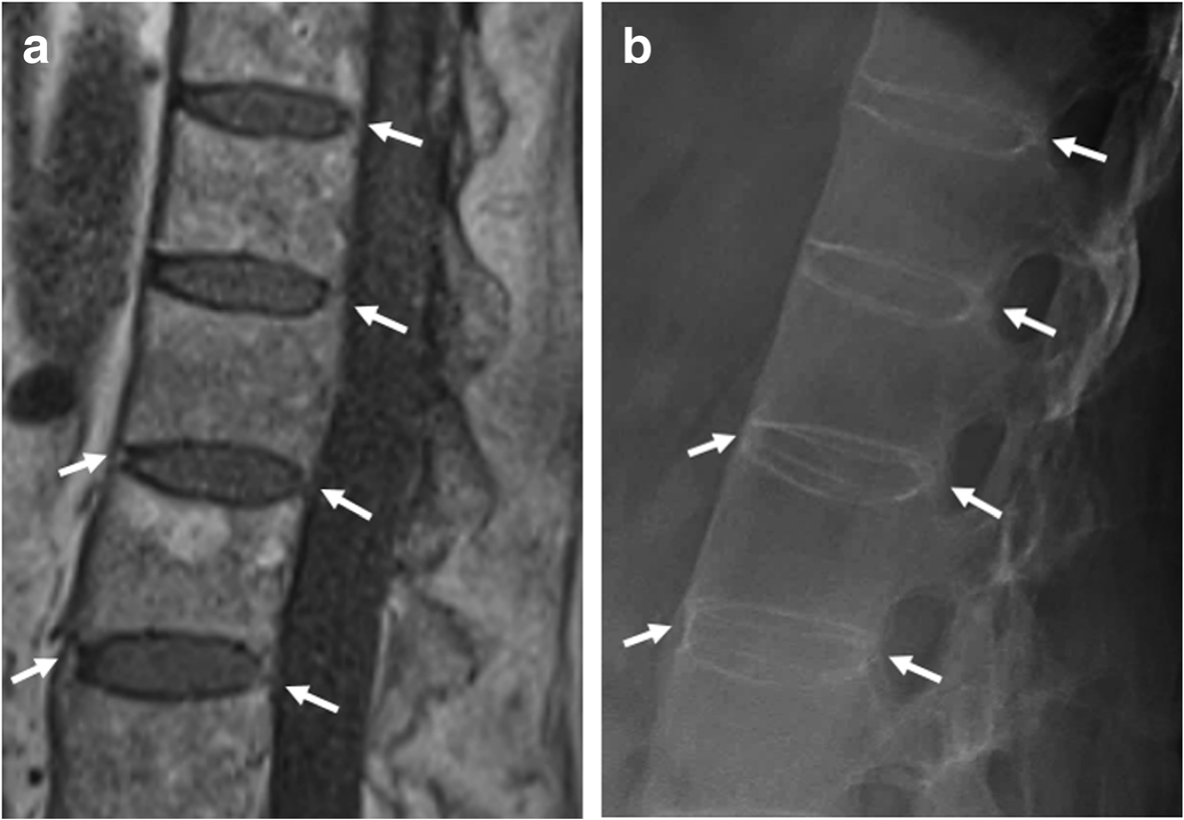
Vertebral corner bridging. a Sagittal T1-weighted MR imaging acquired in a 57-year-old man with SpA shows bridging syndesmophytes (arrows), and (b) radiography of the same vertebrae confirms these vertebral corner bridges (arrows) resulting in a “bamboo spine” configuration. Note that the smaller anterior syndesmophytes are more clearly visualized on radiograph than MRI, which is typical
